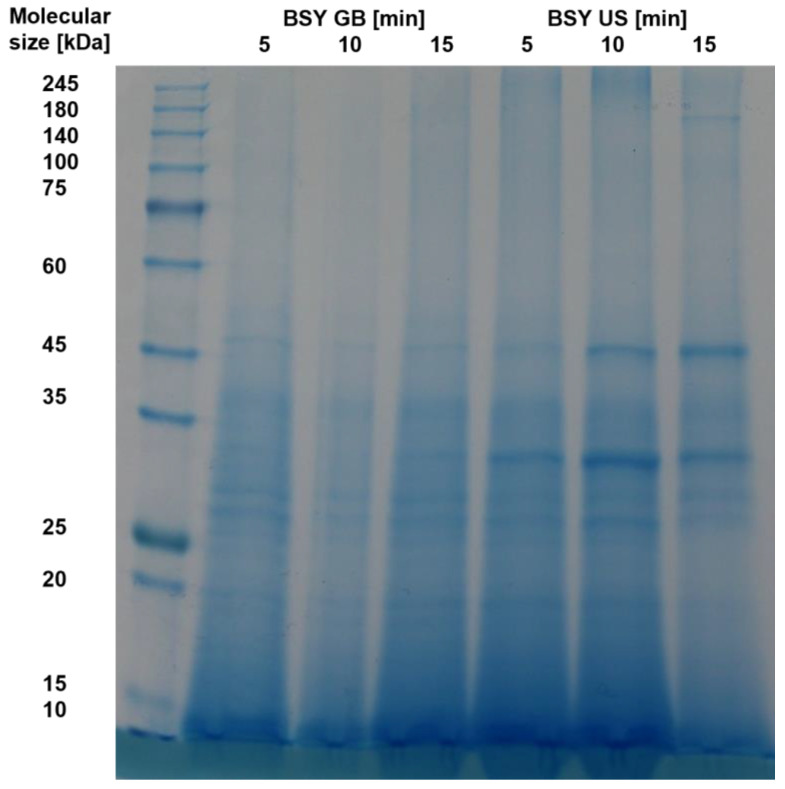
SDS-Page results for glass bead and ultrasound treated BSY extract samples. BSY: brewer’s spent yeast; GB: glass beads; US: ultrasound.
